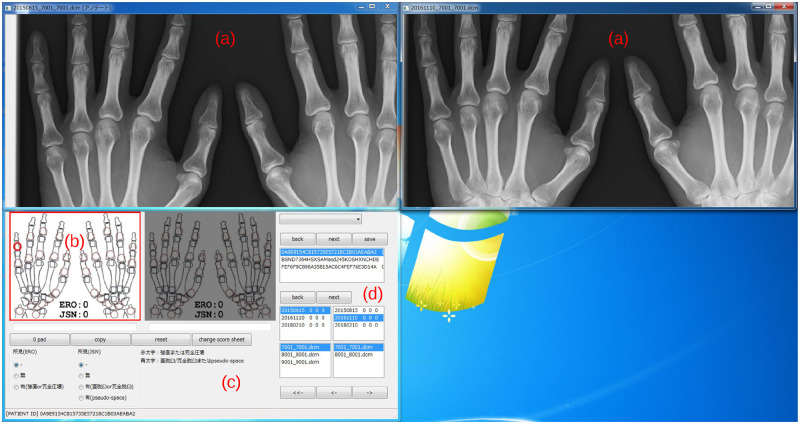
Developed annotation tool for automatic radiographic scoring system. Windows (a) are the image windows that show X-ray images to be annotated, and windows (b) to (d) are the annotation input windows. Since our tool works on the electronic medical record system, the annotators annotated the images during their spare time.
